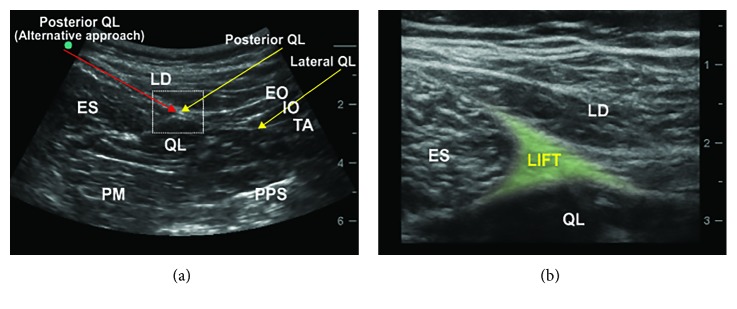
(a) Ultrasound image demonstrating the needle trajectory and tip location of the three different approaches to the ultrasound-guided QL block. (b) Ultrasound image of the lumbar interfascial triangle (LIFT) (ES, erector spinae; LD, latissimus dorsi; QL, quadratus lumborum; EO, external oblique muscle; IO, internal oblique muscle; TA, transversus abdominis muscle; PM, psoas major; PPS, posterior pararenal space).
